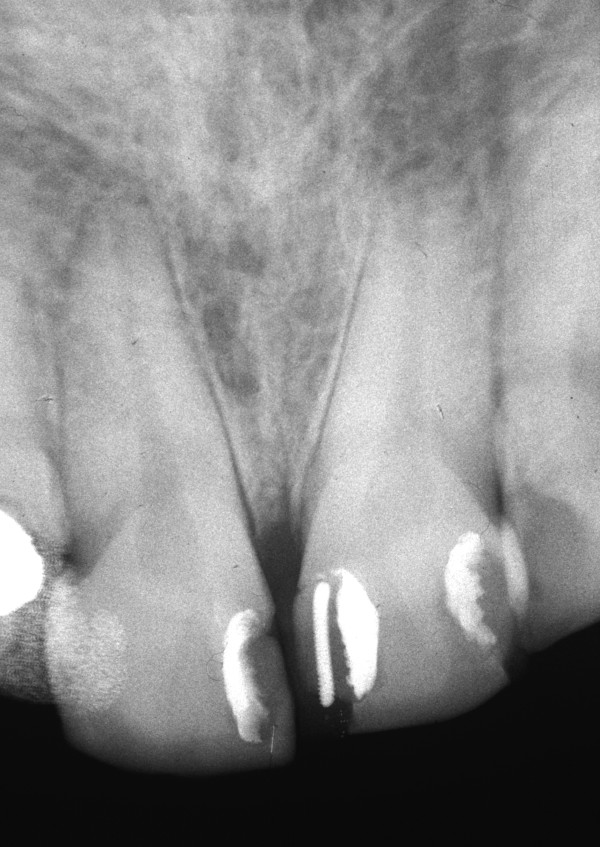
Intra-oral radiograph of the maxillary incisors at time of NUG diagnosis. The angle of the x-ray has shortened the roots.
